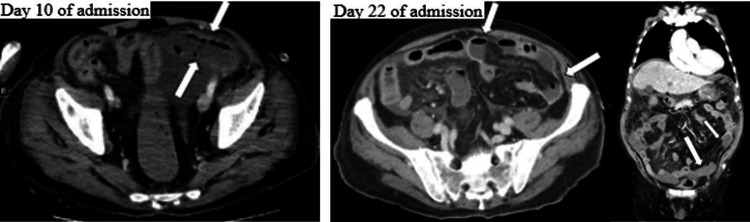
The contrast-enhanced CT images on day 10 and day 22. The tips of the arrows show low-contrast regions. CT, computed tomography
